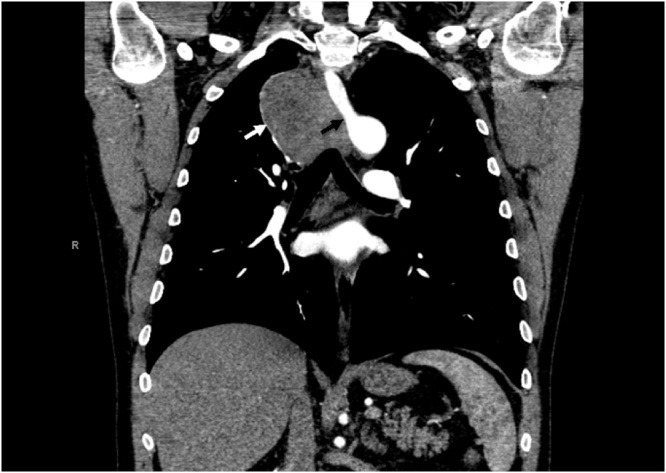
CT demonstrating retroesophageal SCA (black arrow) originating from aorta, medial to esophageal leiomyosarcoma (white arrow).
